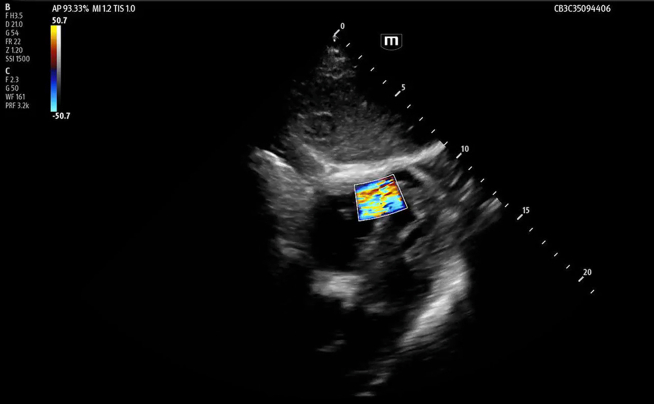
Point-of-care ultrasound revealing ventricular septal defect, apical view.
